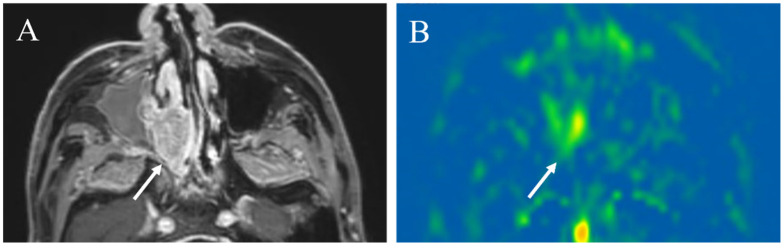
A 87-year-old male with squamous cell carcinoma in the right nasal cavity: (A) Contrast-enhanced T1-weighted image shows an enhanced mass (arrow); (B) pCASL image shows high blood flow within the lesion (arrow) (TBF mean of 70.17 mL/100 g/min) (arrow).
